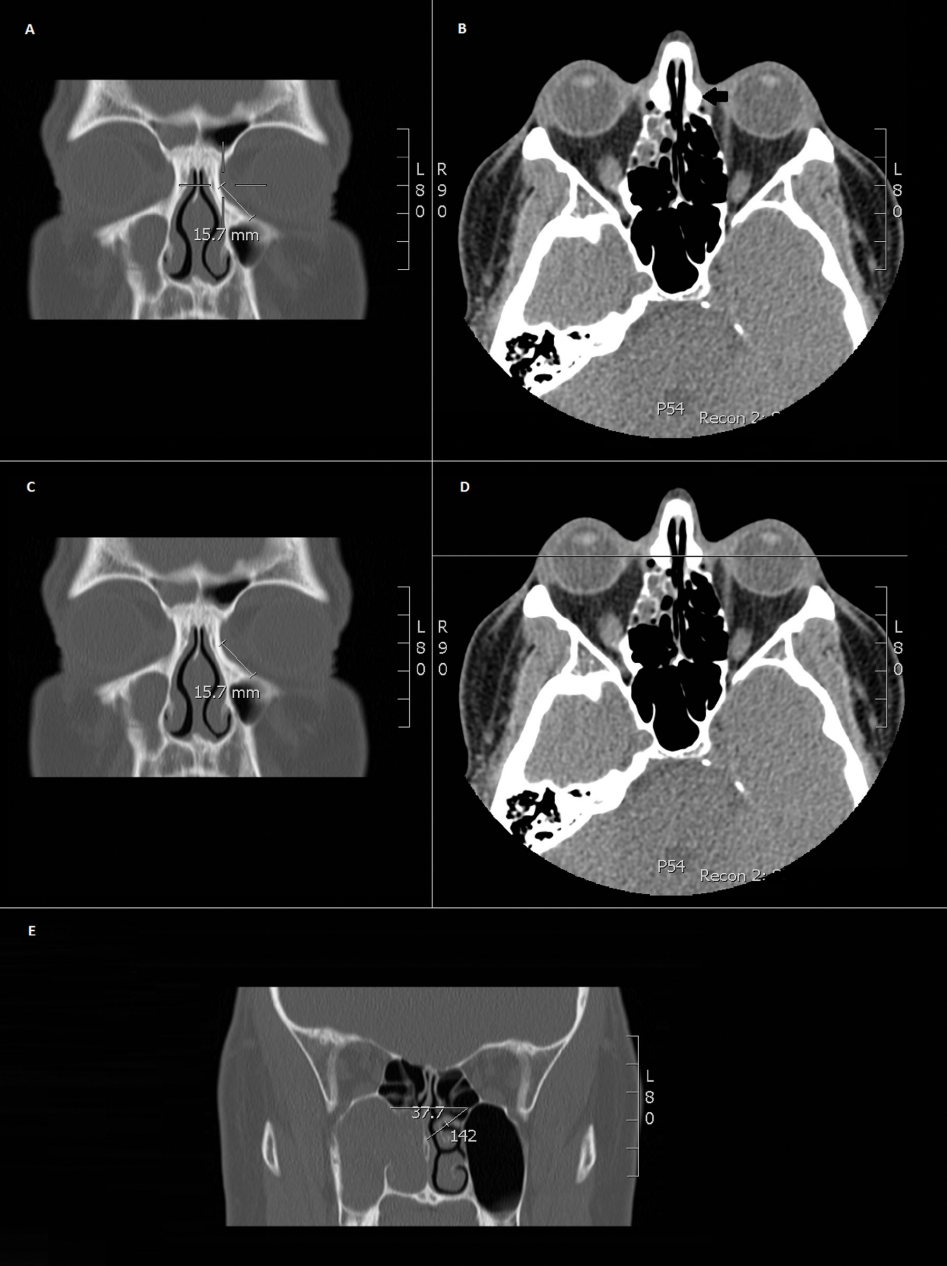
Shows step by step representation of the methodology used to measure the angle. (A) CT scan of the head on coronal cut and (B) axial cut. The black arrow seen pointing at the anterior lacrimal crest on the axial scan with the linked marker seen on coronal cut. (C) and (D) show a diagonal line drawn on the coronal cut, joining the beginning of the anterior lacrimal crest (confirmed by the linked marker on the axial cut) to the end of its curve. (E) Coronal cut of the head showing the horizontal line joining the midpoints of the diagonal lines on either side, with another line going to the most anterior portion of the axilla of the middle turbinate, and the angle present between them (Khedr, Y).
